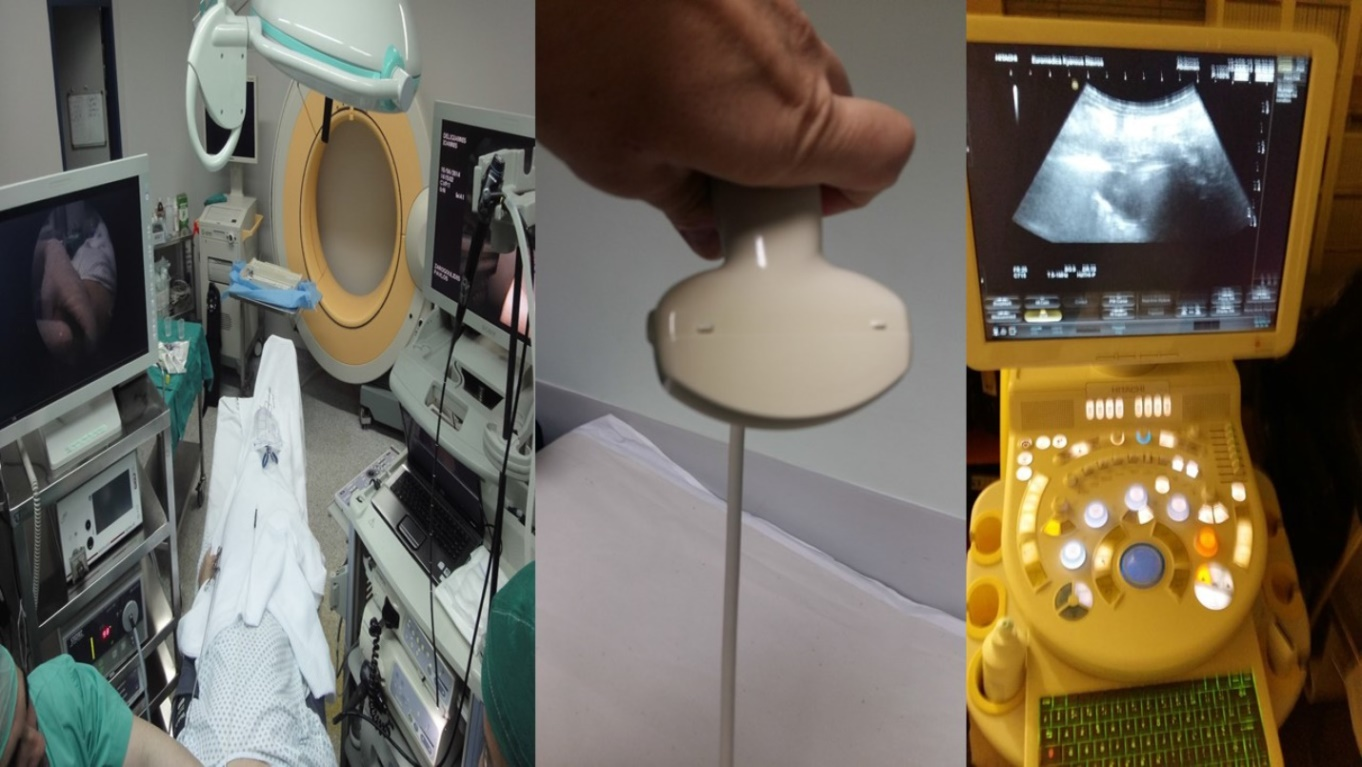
From left to right; Left; O-Arm for navigation, along with rigid and flexible bronchoscope, Middle; convex probe for transthoracic biopsy of superficial thoracic lesions, Right; Ultrasound source for radial, convex probe (EBUS) and transthoracic biopsy.
